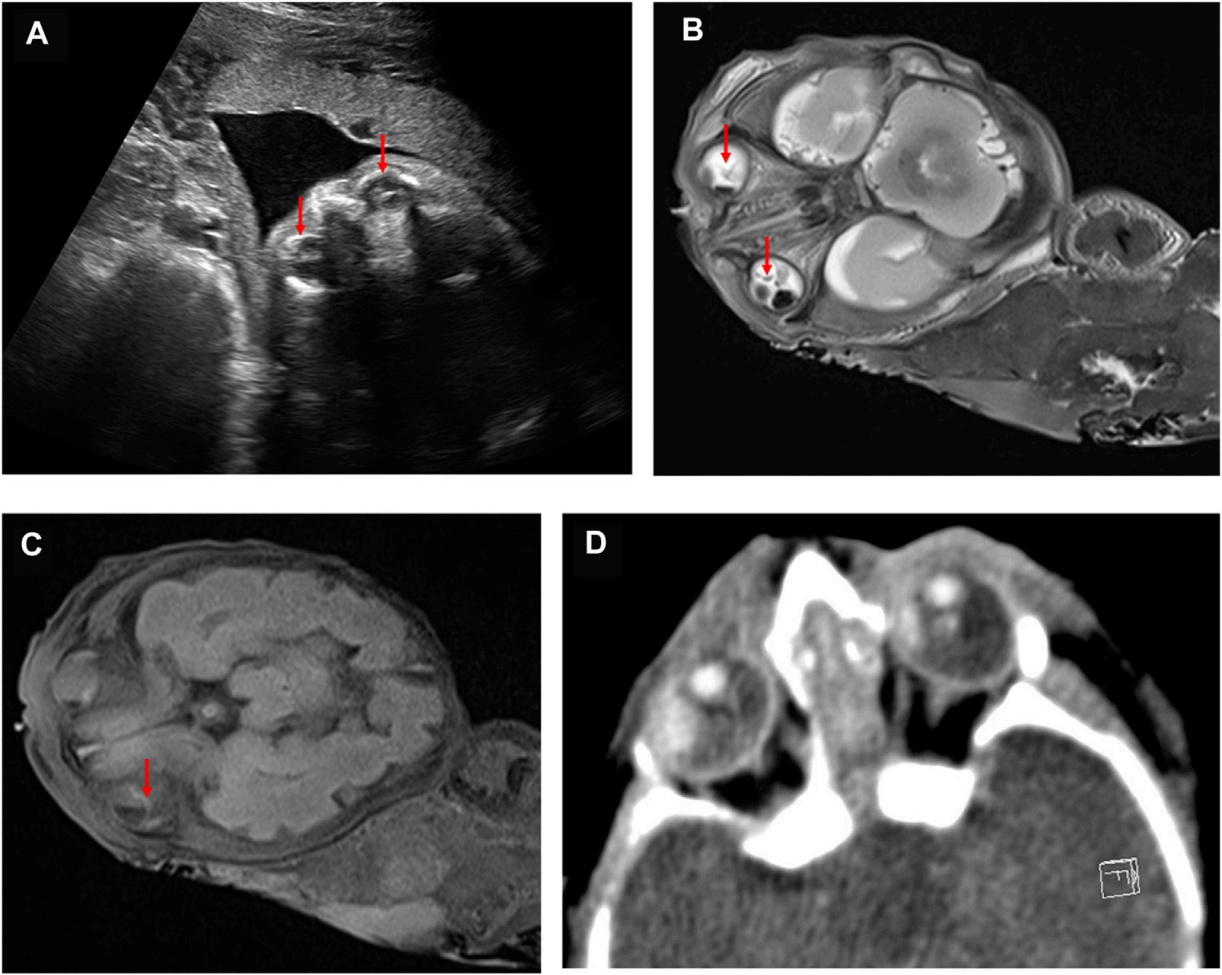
Abnormal fetal imaging results. (A) ultrasound showed a hyperechoic mass in both eyes of the fetus at 25 weeks. (B) MRI showed abnormally enhanced signals in the bilateral vitreous body of the fetus at 27 weeks. (C) as indicated by the arrow, MRI suggests that the fetus at 27 weeks may have a bilateral retinal detachment. (D) CT scan showed abnormal bilateral enhancement of the eyeballs in the stillborn infant.
